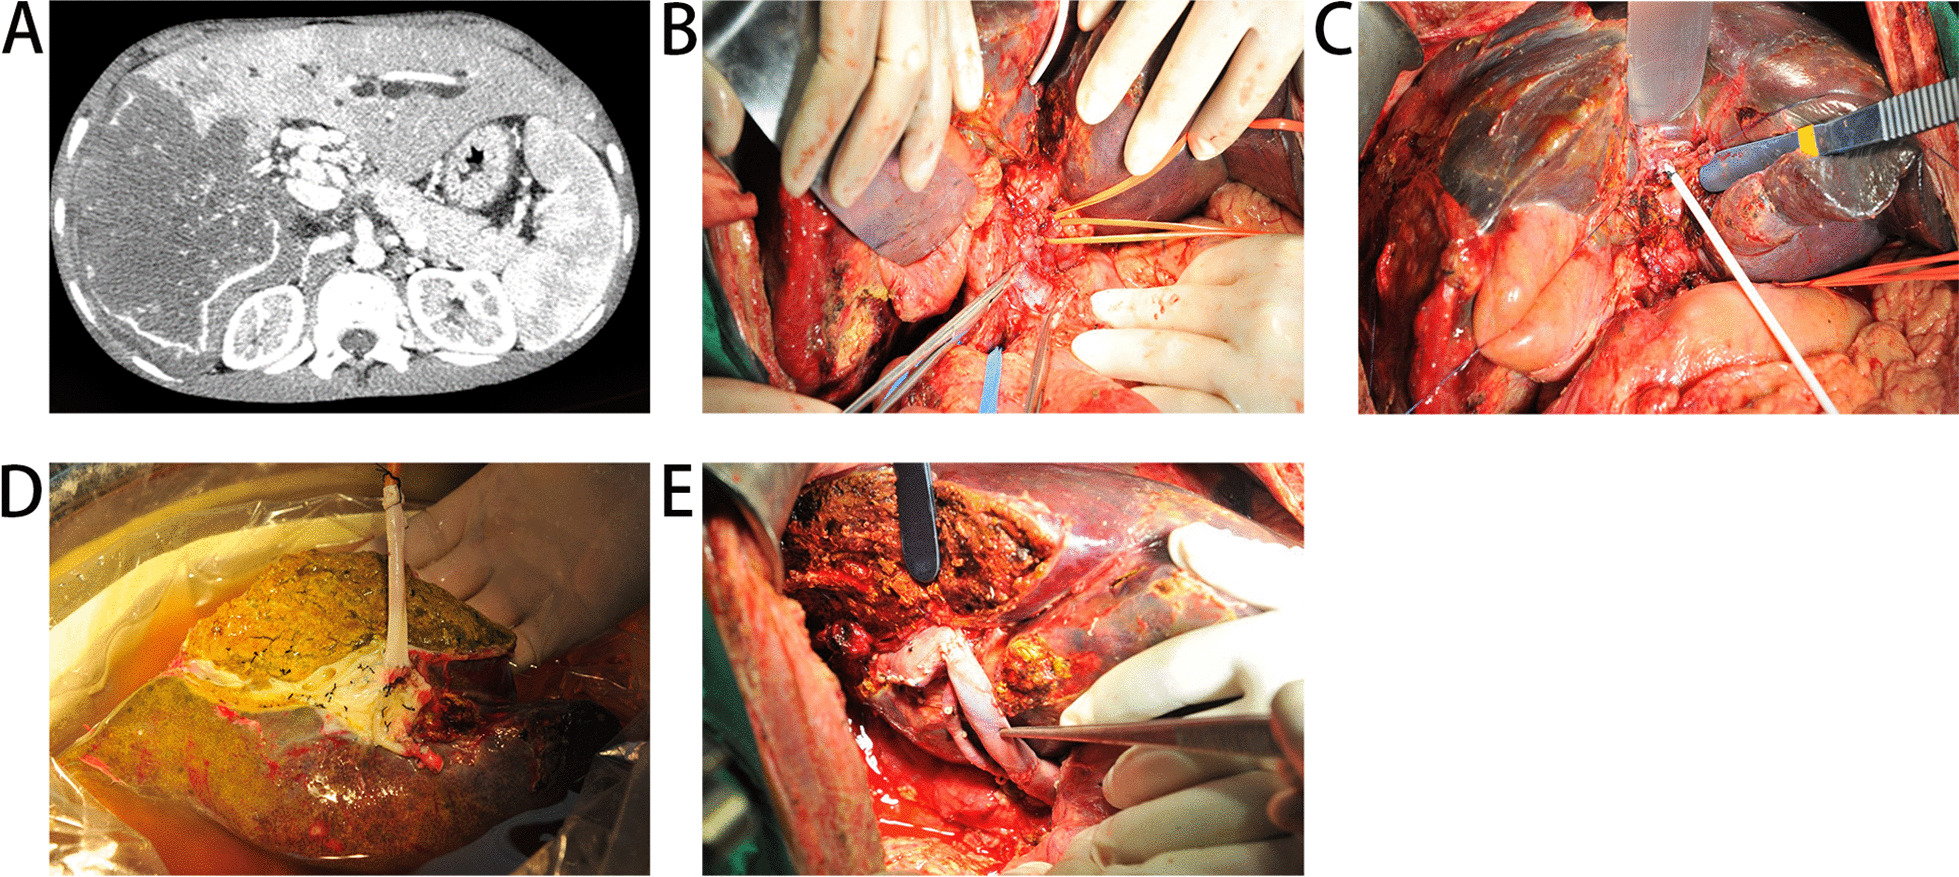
treatment of portal cavernous variant by ELRA: A CT image of hepatic vesicular hydatid induced portal cavernous degeneration. B Varicose vessels are seen in the hilar area. C The round ligament of the liver is catheterized to guarantee extracorporeal perfusion of the liver. D reconstruction of the portal vein with blood vessels in patients after cardiac death E anastomosis of the portal vein
